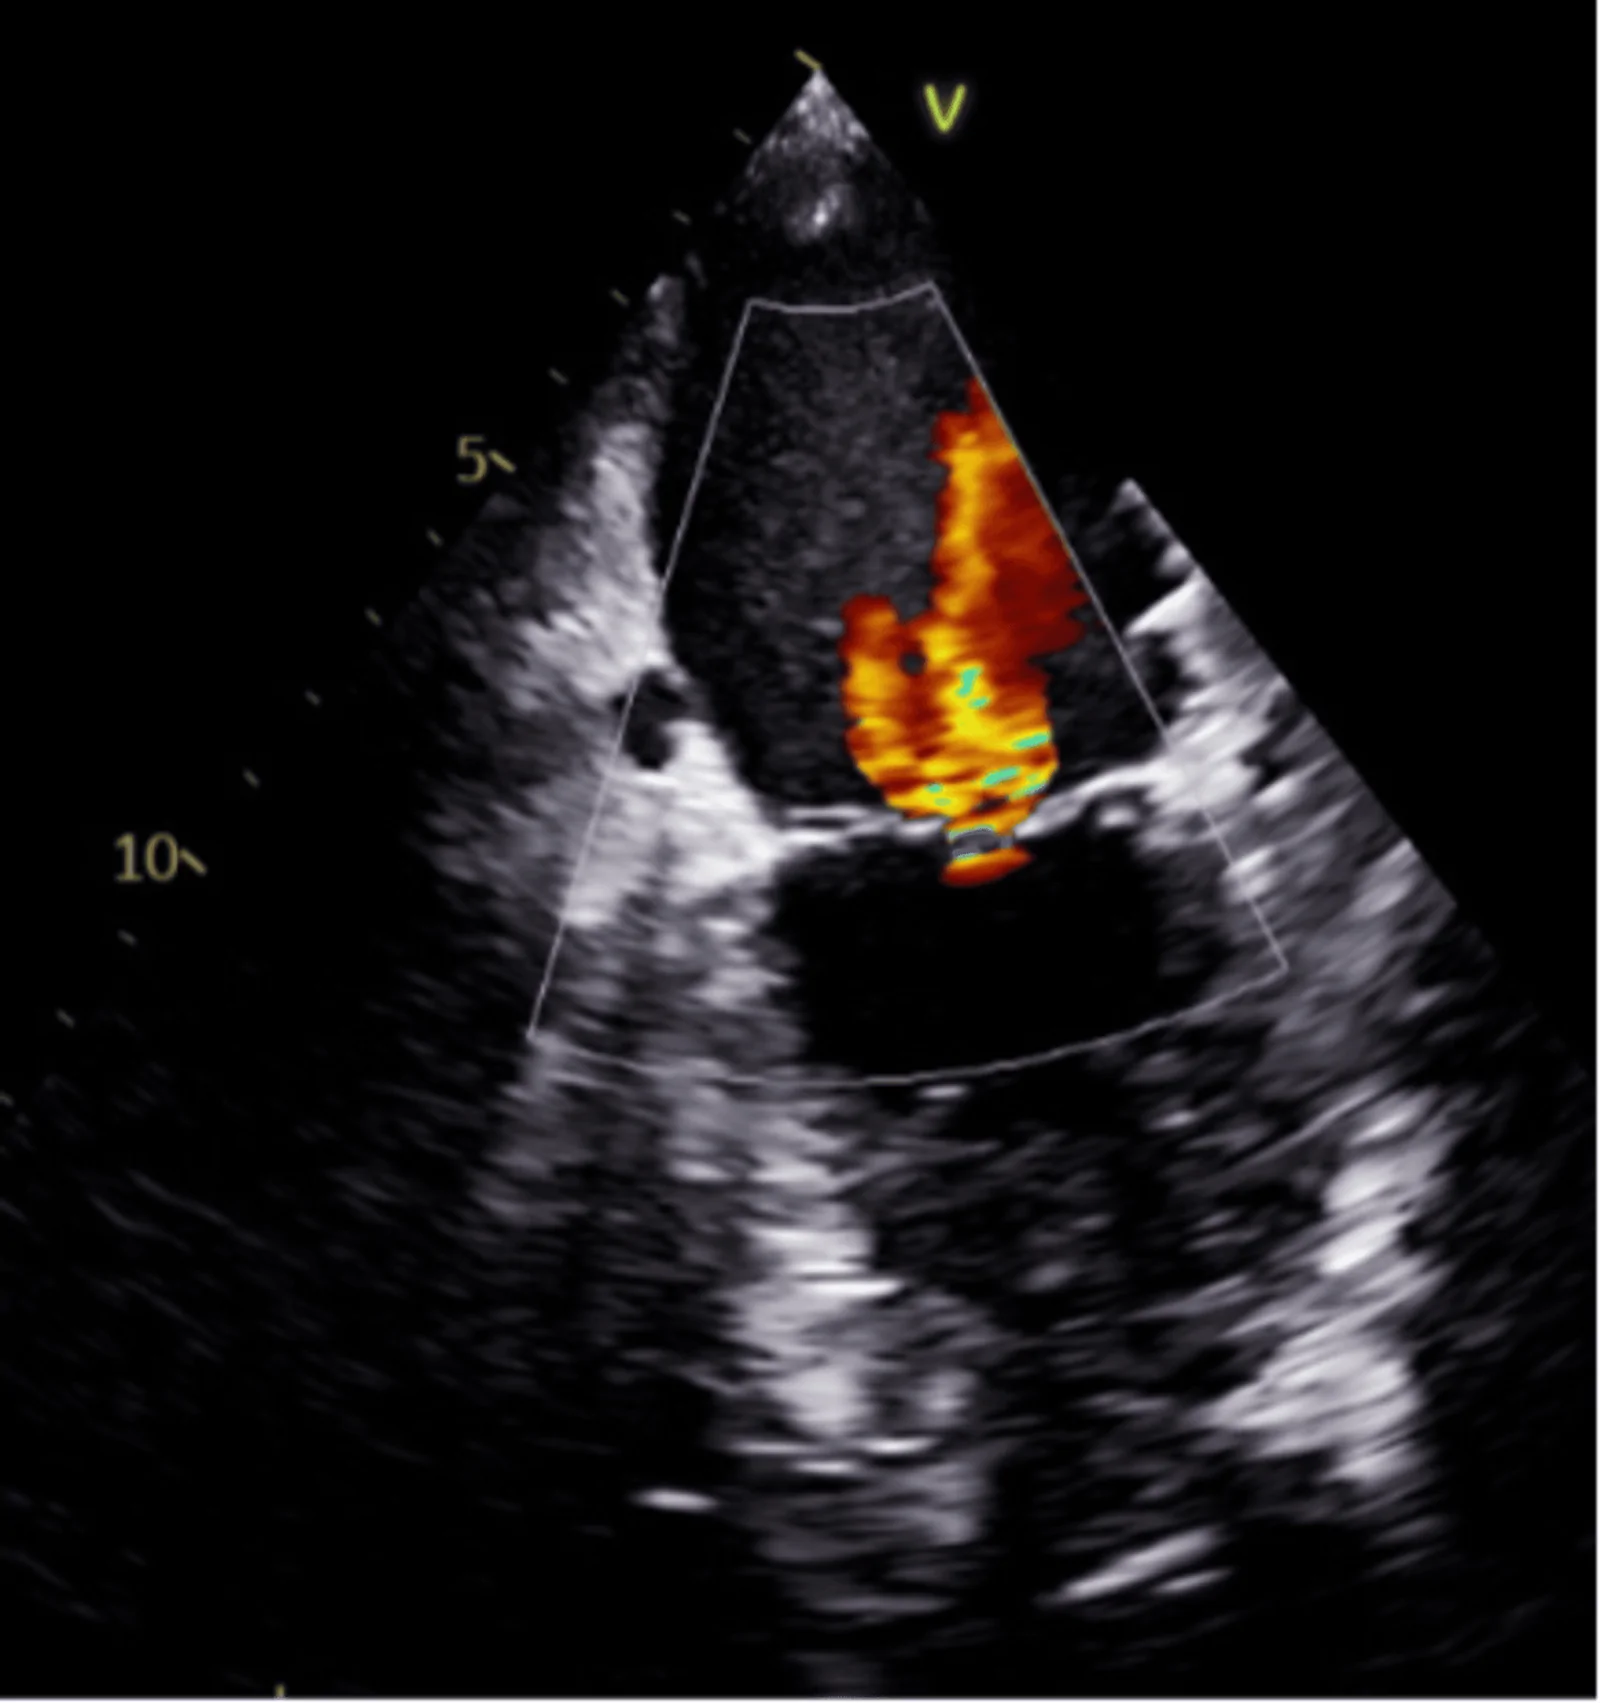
Mitral regurgitation. Color Doppler TEE image showing mitral regurgitation, demonstrated by a regurgitant jet extending into the left atrium. TEE, transesophageal echocardiography
